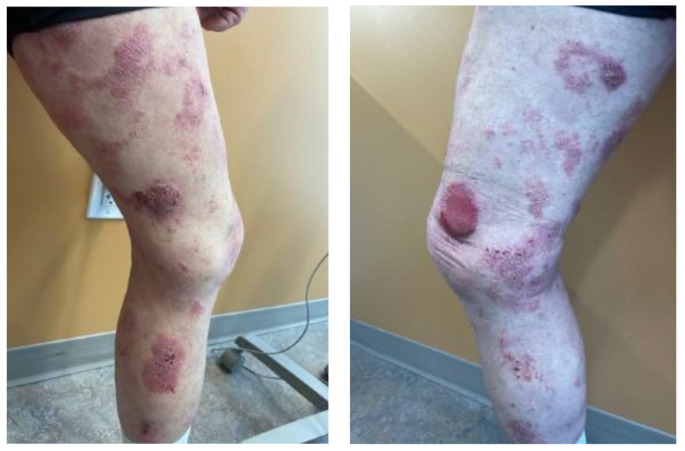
Skin manifestation. Extensive involvement of CTCL with tumor, plaques and patches affecting bilateral lower legs. Thick plaques and patches (left panel) shown on the right leg. Thick eroded tumor present on the right anteromedial knee (right panel) showed maximal uptake on the PET-CT scan (Figure 3).
